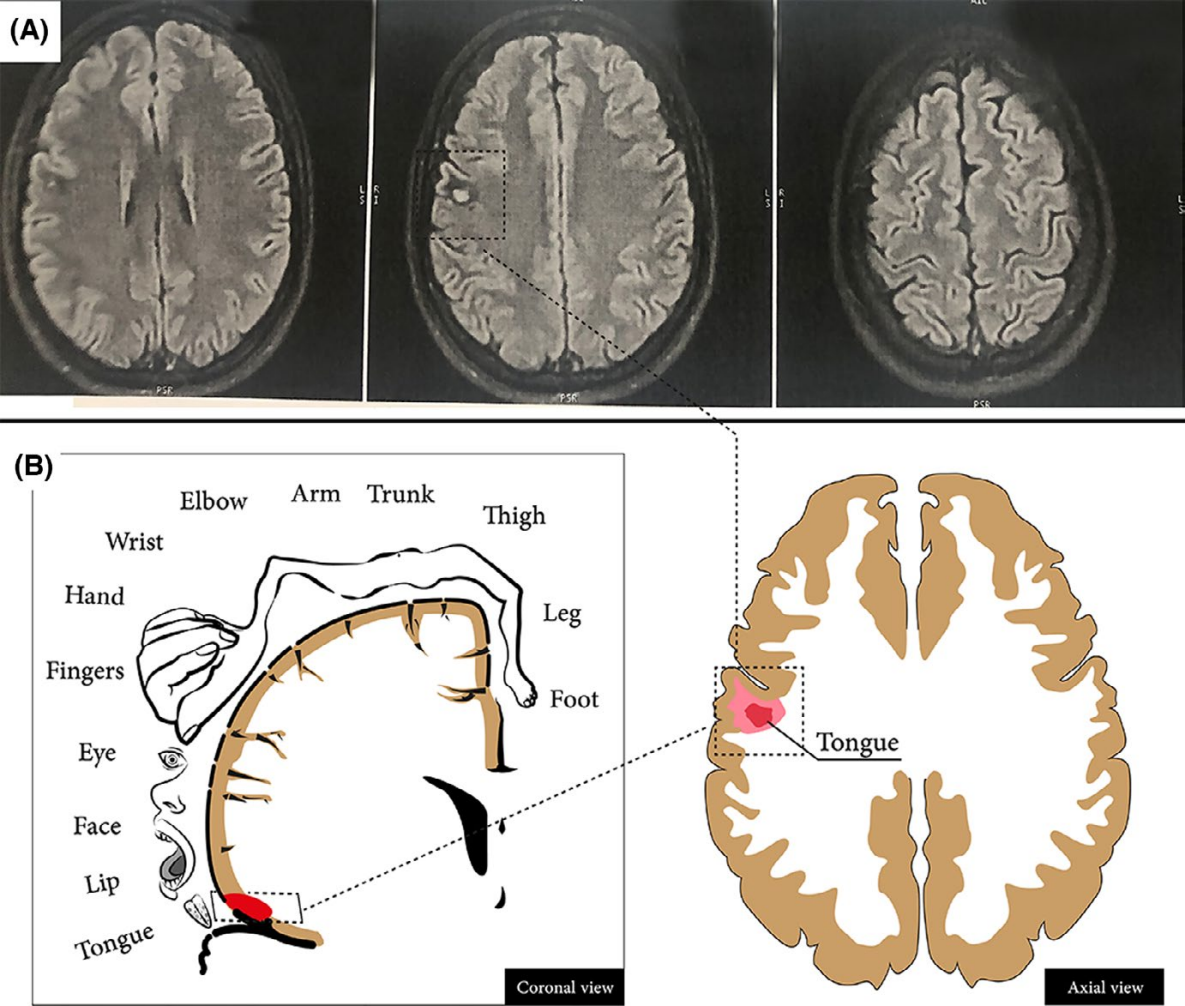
(A) Axial FLAIR imaging demonstrated focal hyperintensity with hypointense suggesting cavernous angioma. (B) Homunculus presentation of the tongue demonstrated the involvement of the tongue motor cortex
